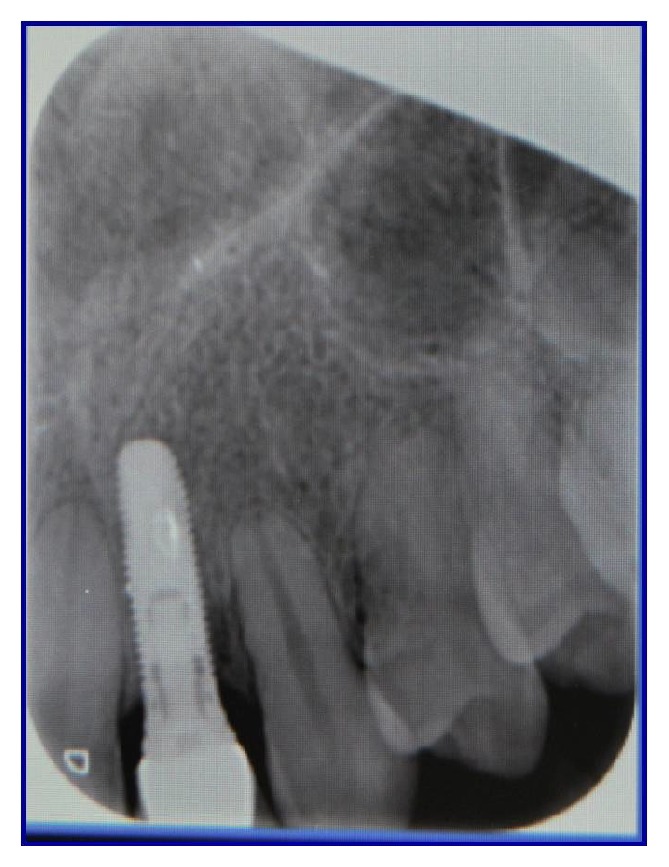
Radiographic control after one year in site to the right.
